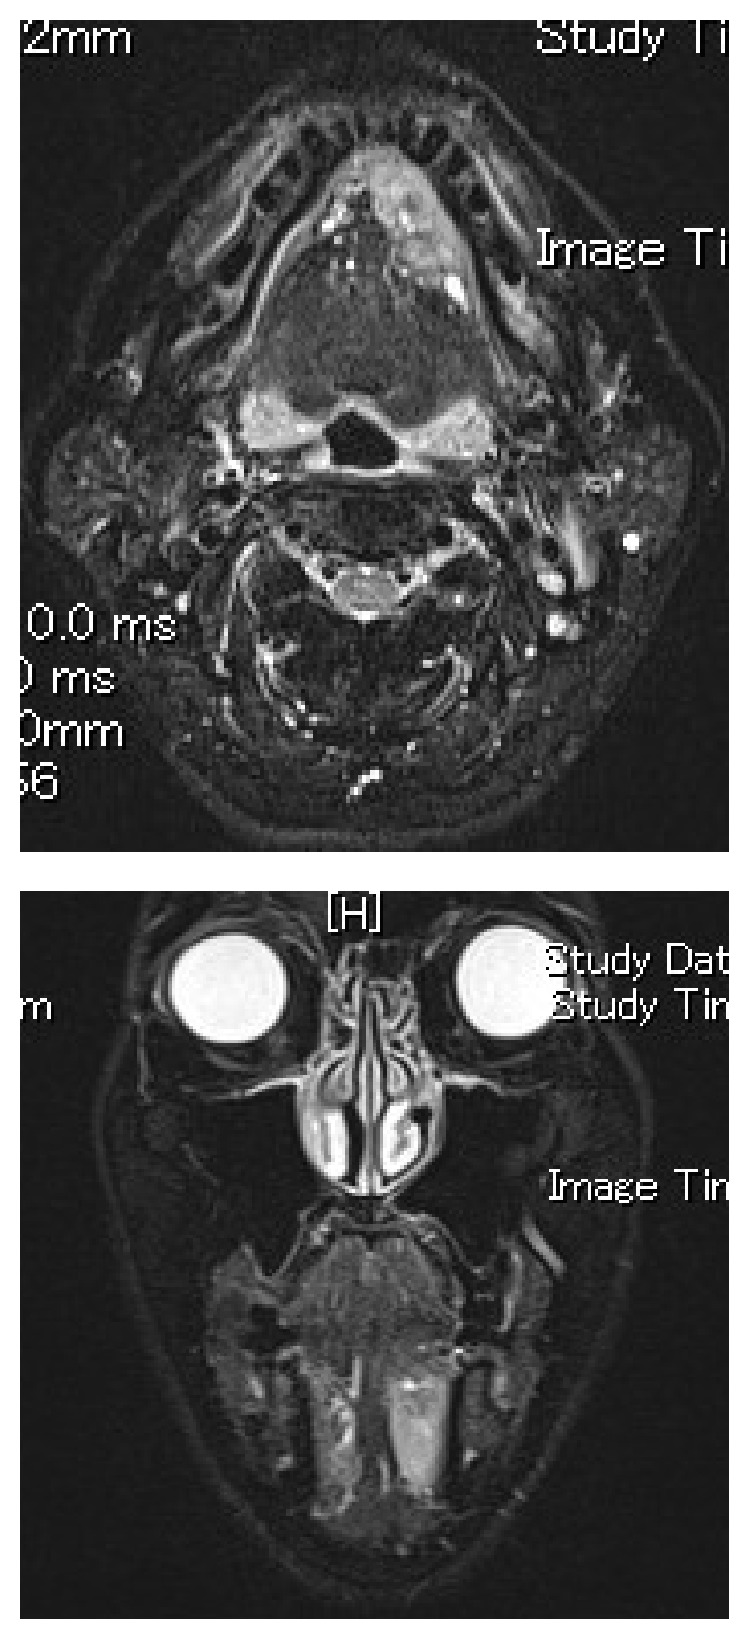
MR images (STIR) showed a well-defined tumor of the sublingual gland with a high-signal mass measuring 28 × 25 × 12 mm.
